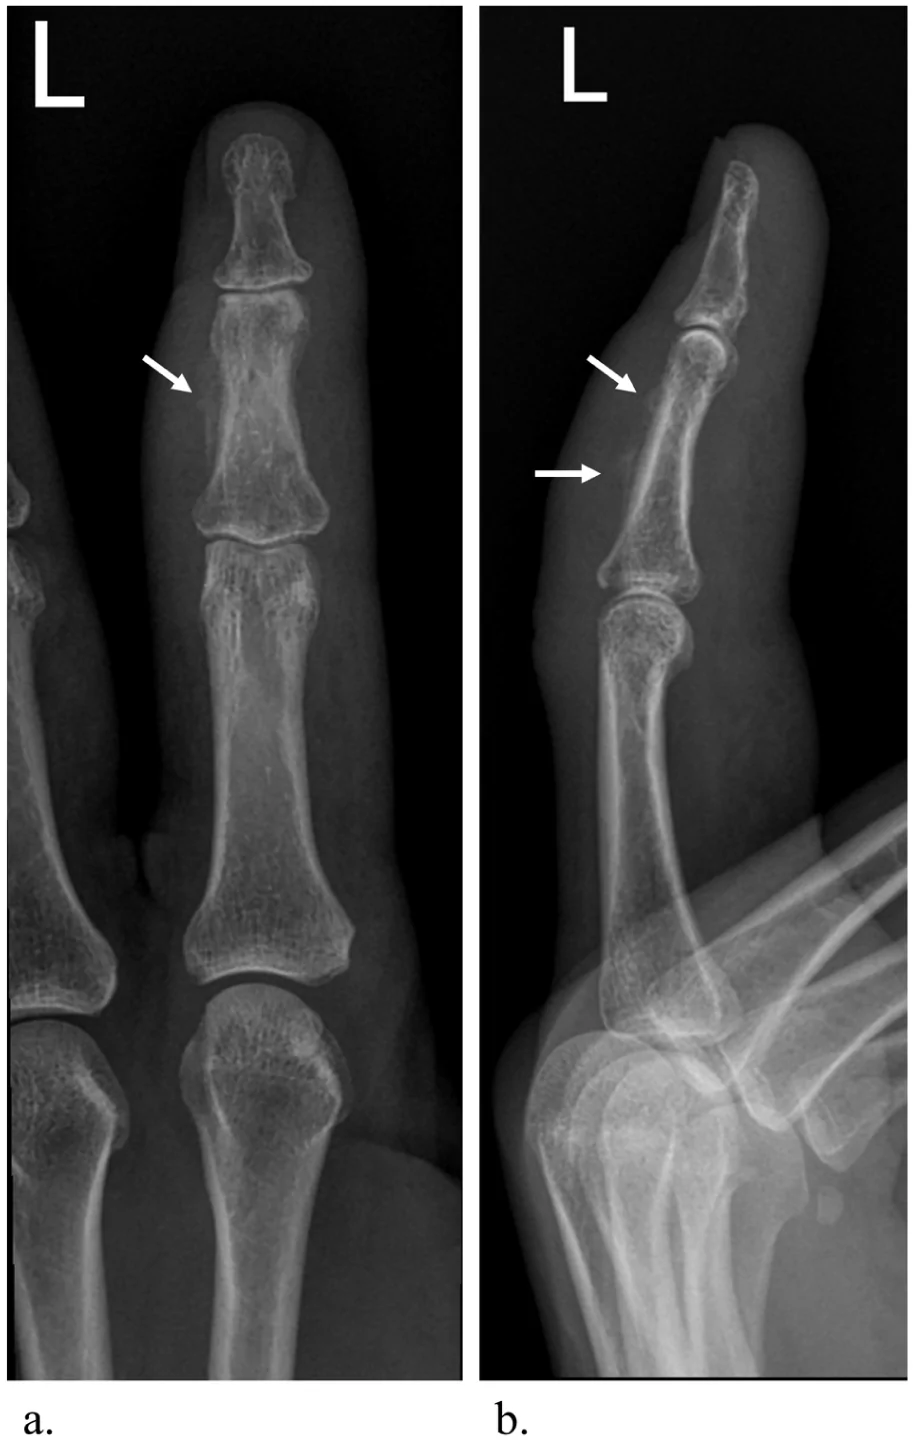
Left index finger radiographs ((A) posteroanterior and (B) lateral) 4 weeks post initial excision of fibro-osseous pseudotumour of the digit, with recurrent soft-tissue swelling. Focal intralesional/periosteal mineralisation (white arrows) tracking along the dorsal/ulnar cortex of the mid to distal shaft/neck of the second middle phalanx with significant overlying soft-tissue swelling. No erosion of the cortex or abnormality interphalangeal joints on radiography.
